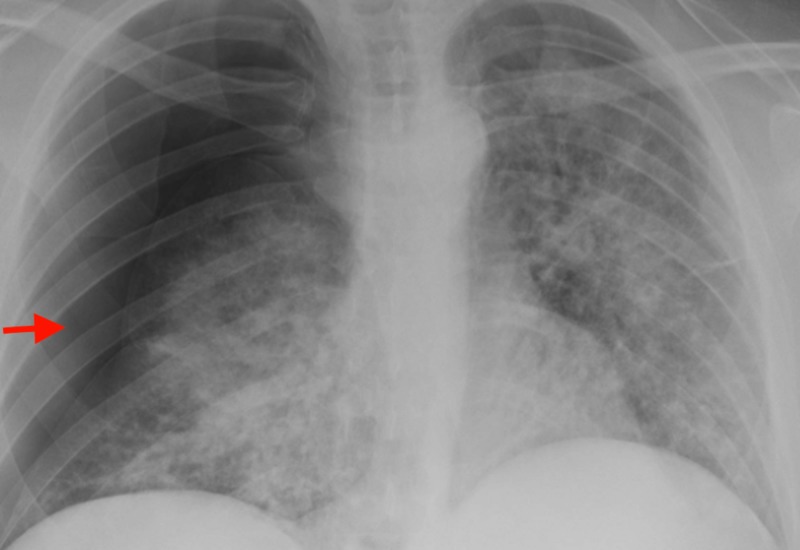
Chest X-ray showing right-sided pneumothorax with slight shift of cardiac and mediastinal structures to left.
